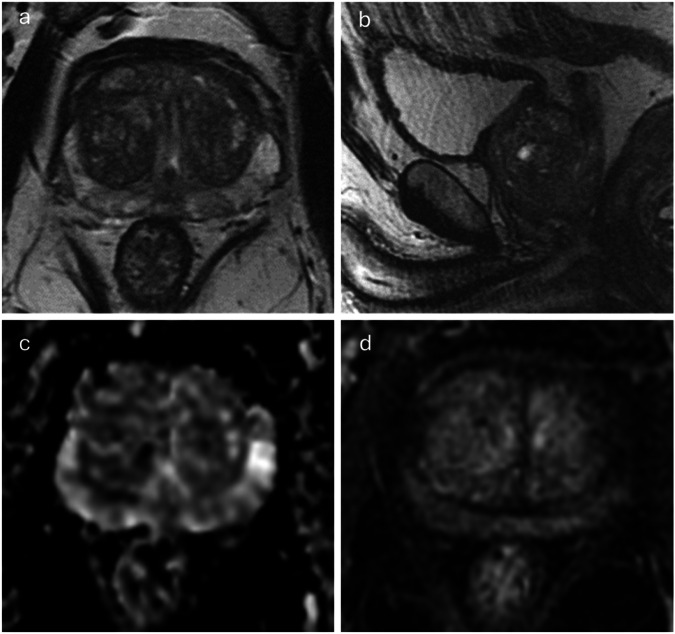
Image quality categorisation varied between versions because of sagittal T2WI, overall image quality was classified as optimal on PI-QUAL v1 compared to acceptable on v2 bpMRI and v2 mpMRI. Image of a 76-year-old patient scanned at 3-T MRI with a presenting PSA of 5.24 ng/mL and a prostate volume of 70 cc (PSA density: 0.07 ng/mL/mL). According to PI-QUAL v1, the axial T2WI (a), DWI (c), and DCE (d) sequences all exhibit optimal quality, resulting in an overall v1 quality score of 5/5, equivalent to optimal image quality (3/3) on a 3-point scale. However, under PI-QUAL v2, while the DWI (4/4) and DCE (2/2) sequences receive full marks, the sagittal T2WI (b) is not considered optimal, resulting in a T2WI score of 3/4, and an overall mpMRI image quality score of acceptable (2/3)
